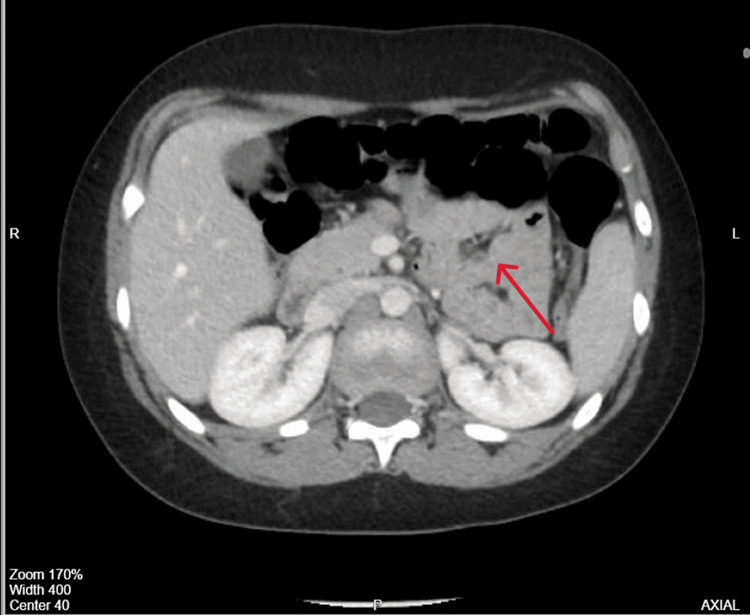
Computed tomography (CT) scan showing ileoileal bowel intussusception with multiple enlarged lymph nodes nearby. There is no free fluid or abdominal collection. The solid organs are unremarkable.
